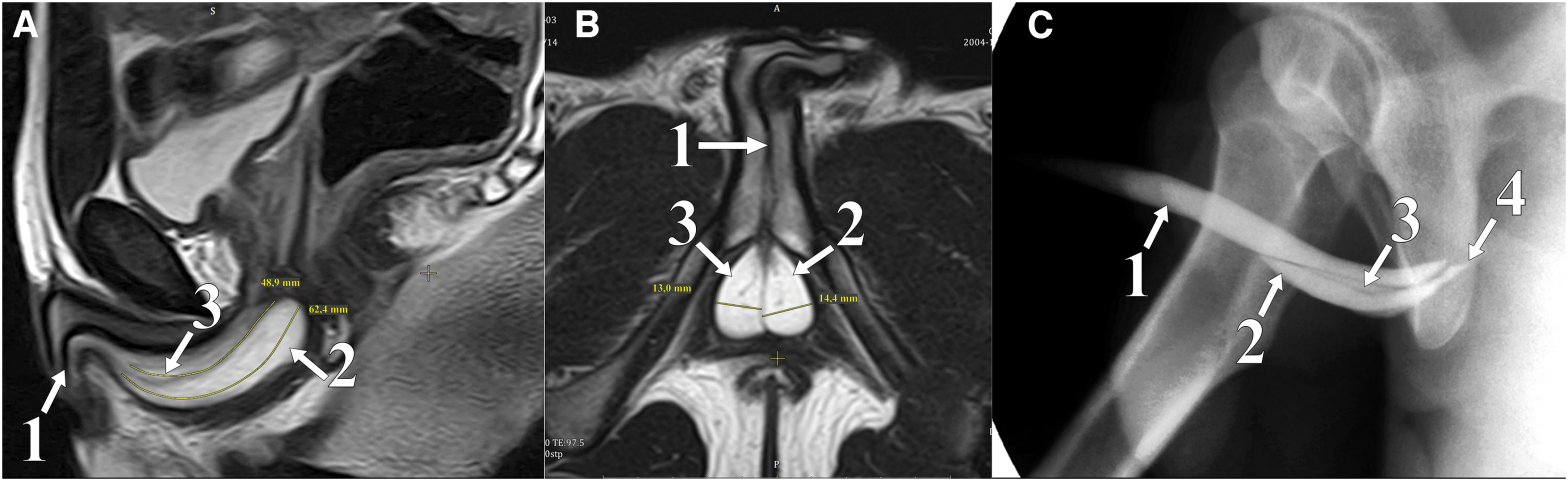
Lateral (A) and sagittal (B) plane MRI: T2 WI mode; soft tissue in the pelvis. Retrograde urethrogram (C): two paraurethral cavities in the bulbar area contrasted. (1) Urethra, (2) left chamber CS, (3) right chamber CS, and (4) Cowper's duct.
